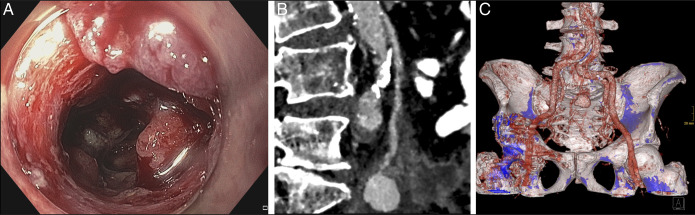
An 86-year-old man without significant comorbidity presented with weight loss, diarrhea, and iron deficiency anemia. No signs of gastrointestinal bleeding were noticed. Colonoscopy was performed, and sigmoid cancer was suspected (a). Biopsies were obtained, which confirmed colonic adenocarcinoma. Eight hours after the endoscopy, the patient developed massive hematochezia, followed by hemodynamic instability (blood pressure 60/30 mm Hg; heart rate 120 bpm; and hemoglobin drop from 9.8 g/dL to 6.1 g/dL within 2 hours). After stabilization with intravenous fluids, norepinephrine, and transfusion of 1 unit of red blood cells, endoscopy was repeated and ruled out ongoing bleeding. A computed tomography scan confirmed a mass of the sigmoid colon, which extended along the inferior mesenteric artery and to the os sacrum. Within the sigmoid wall, extravasation of the contrast medium was seen into a structure of 2.3 × 1.7 cm. Computed tomography reconstructions suspected pseudoaneurysm of the inferior mesenteric artery (Figures B, C). In the absence of distant metastases, surgical resection was attempted urgently. However, owing to infiltration of the aortic wall, curative resection was impossible and colostomy was performed. Angiography confirmed a large pseudoaneurysm of the rectal superior artery, and embolization was performed successfully. The further course was unremarkable.
